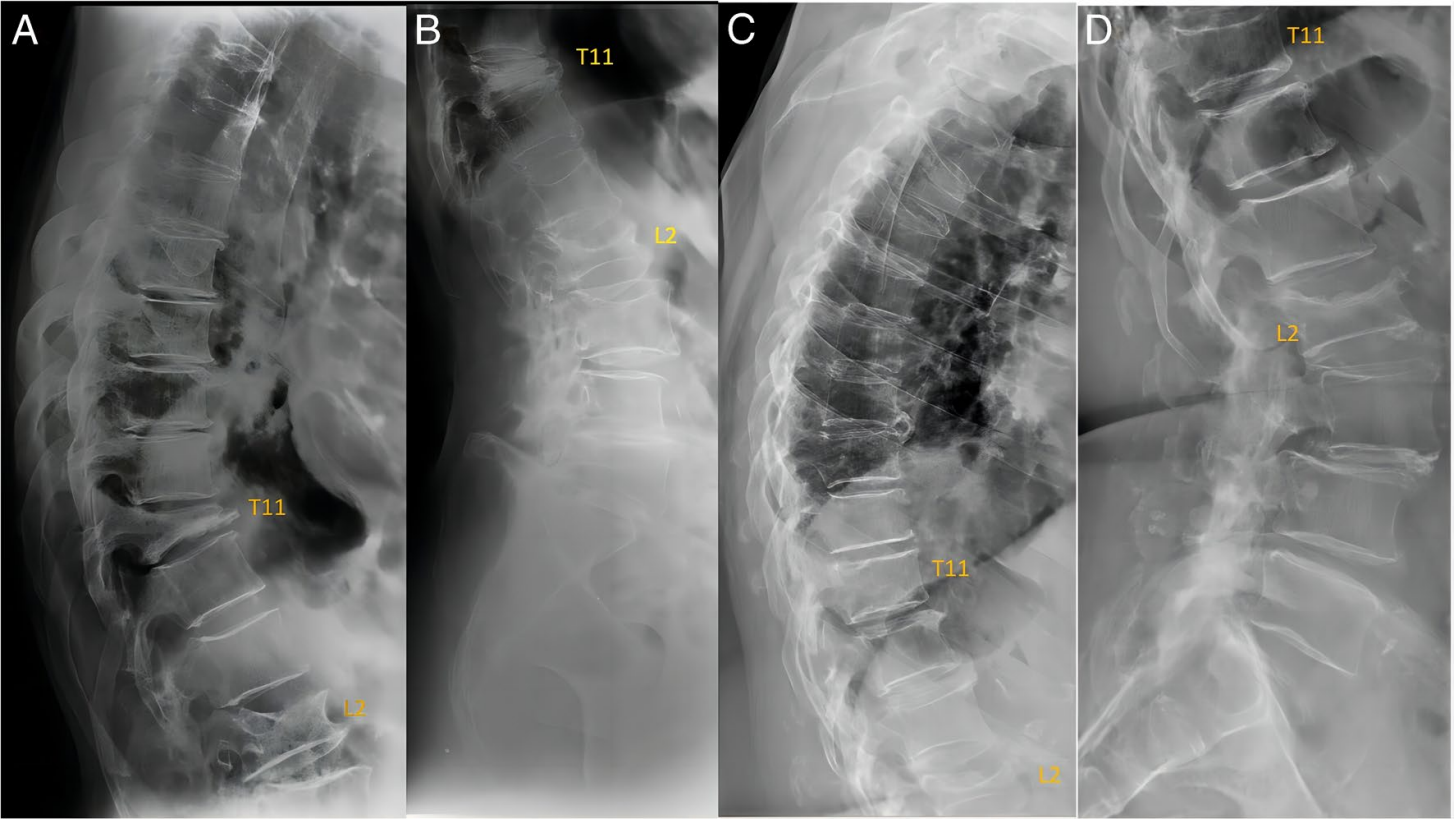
The X-ray projection has important implications in the appearance of vertebrae. A and B are from an elderly woman, and (C) and (D) are from a different elderly woman. A and B show vertebra collapse of T11. Note the extent of height loss at L2 appears to be more severe in B than in A. It is likely that L2 is more “off-center” with respect to the X-ray beam focus in (B) than that in (A). The morphology of the superior endplate of T11 mimics a depression on D, while on (C) the superior endplate of T11 appears to be normal. Note the extent of height loss at L2 appears to be more severe in (C) than in (D). It is likely that L2 is more “off-center” with respect to the X-ray beam focus in (C) than in (D). Reproduced with permission from Wáng. Quant Imaging Med Surg. 2022;12:3495–3514
